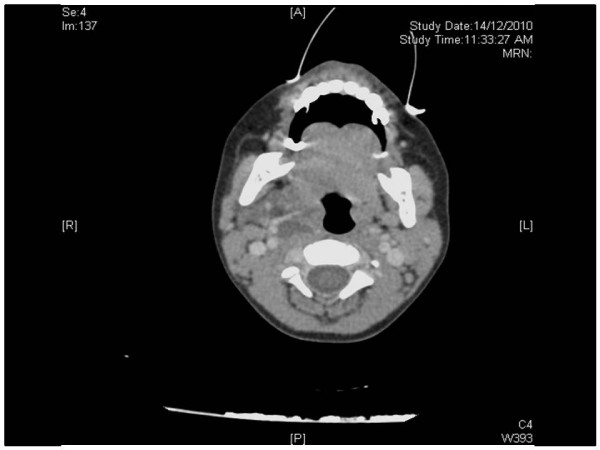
Post operative computed tomography (CT) scan imaging showing multiple fluid containing rim enhancing lesions in the right side of neck.
